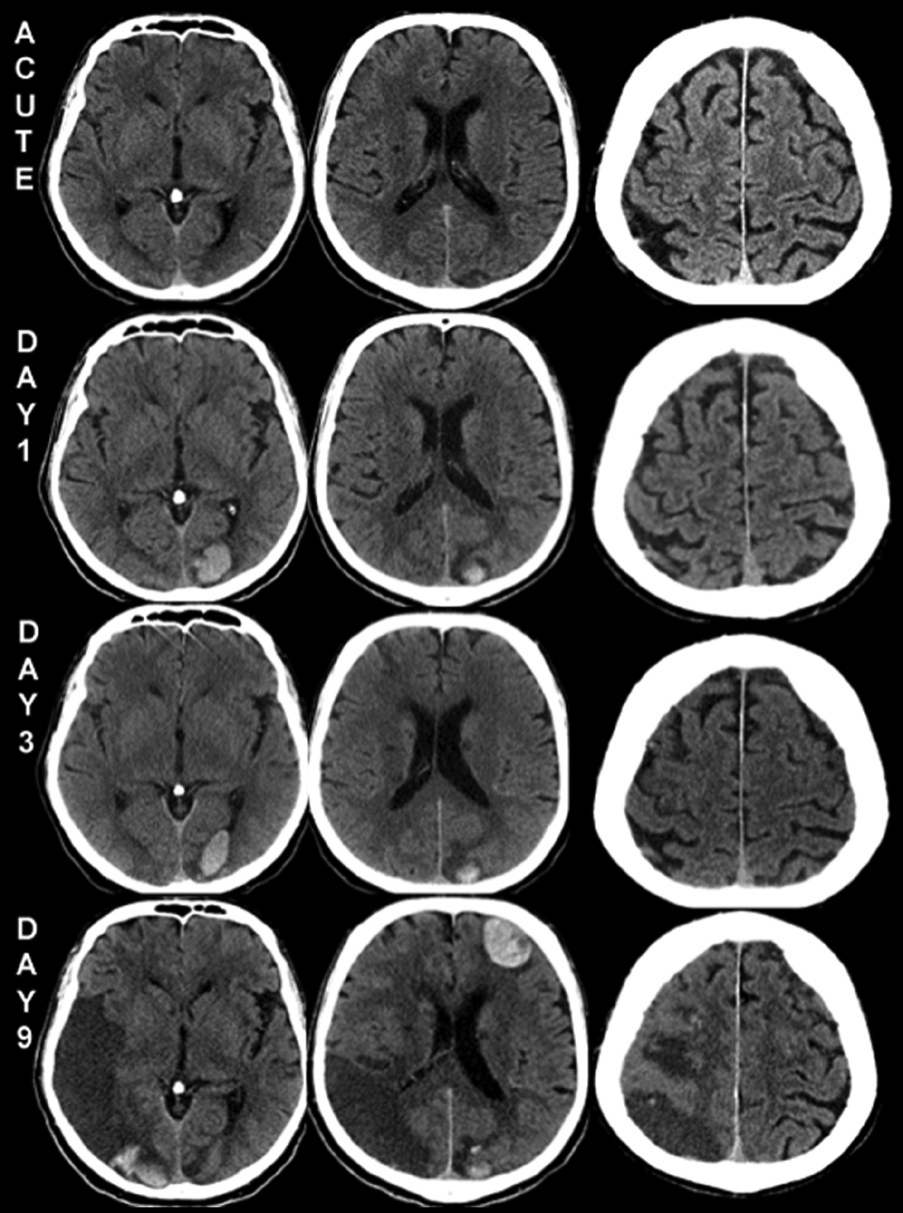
Multiple widely spread cerebral infarctions developing over the course of 9 days. Acute CT scan showed a number of small infarctions. Additional CT scans day 1, day 3, and day 9 revealed several new and larger infarctions with hemorrhagic transformations on both sides.
